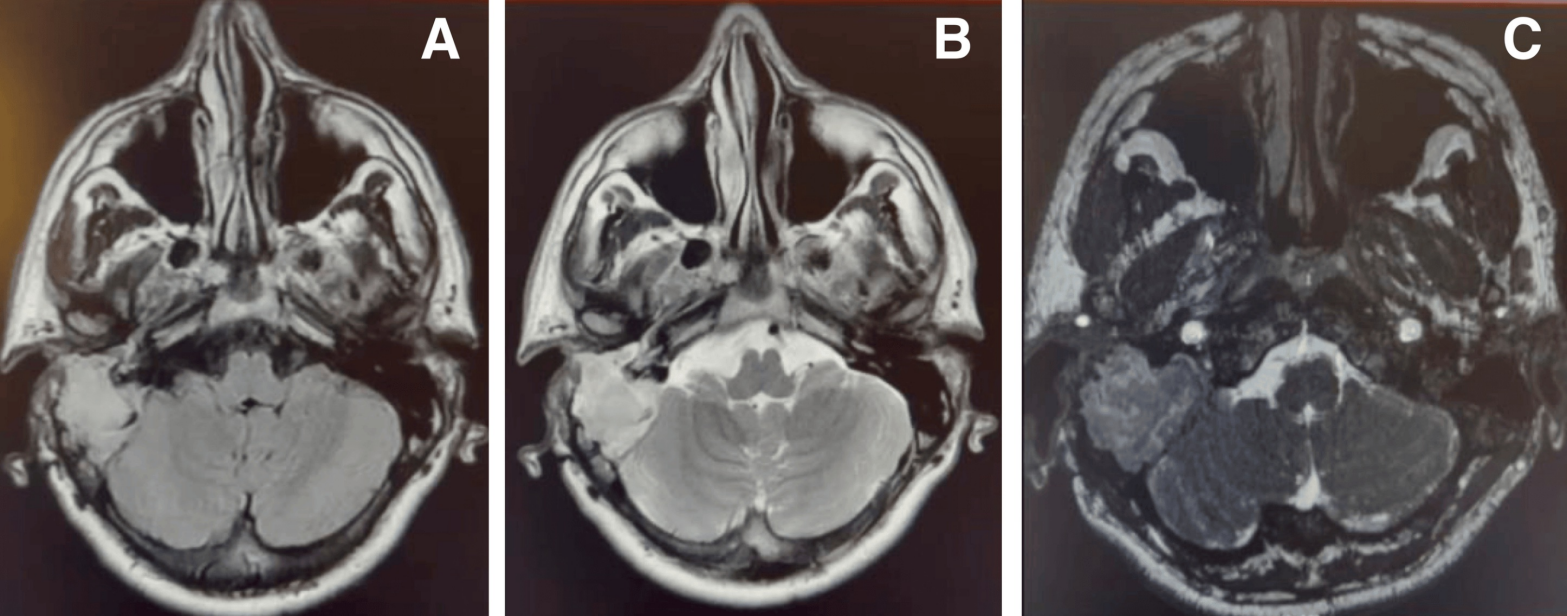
The preoperative MRI shows soft tissue intensity of the right mastoid air cells with encasement of the distal jugular vein and thinning of the tegmentum tympani. A: T1 without contrast; B: T2 without contrast; C: T1 with contrast
